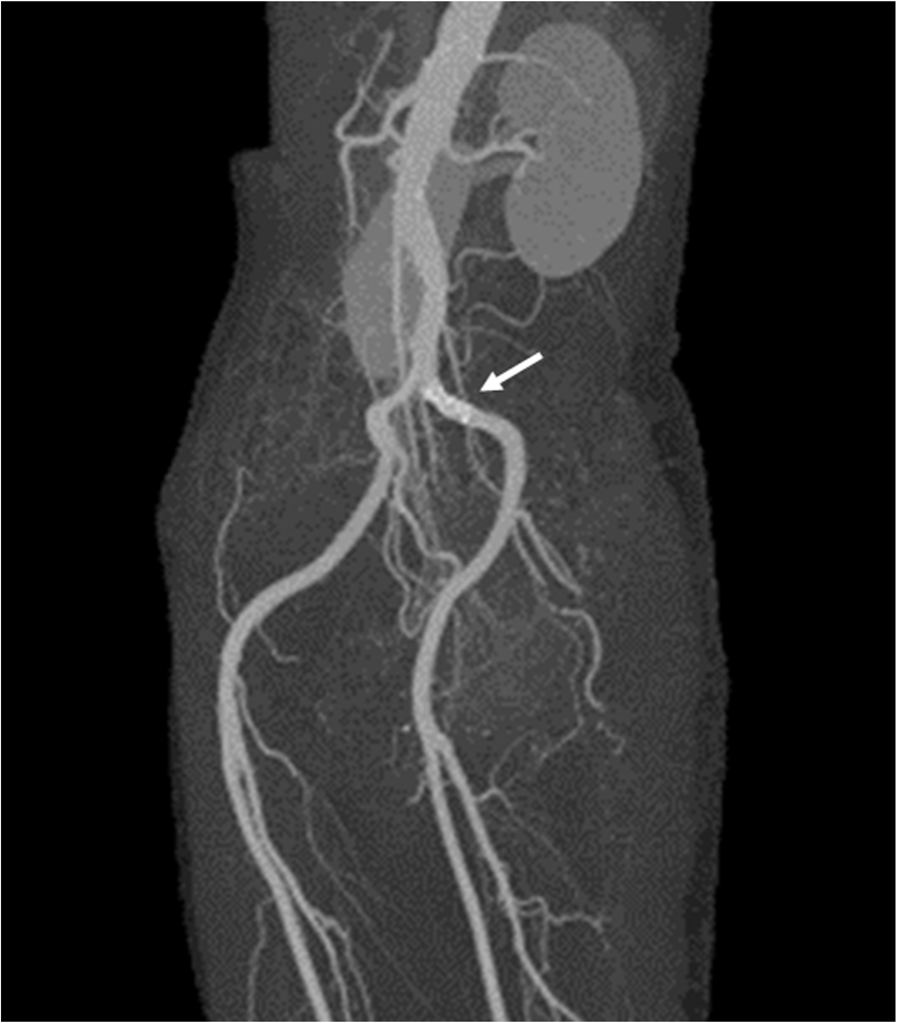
On contrast-enhanced computed tomography the stent (arrow) implanted in the left common iliac artery showed good patency
